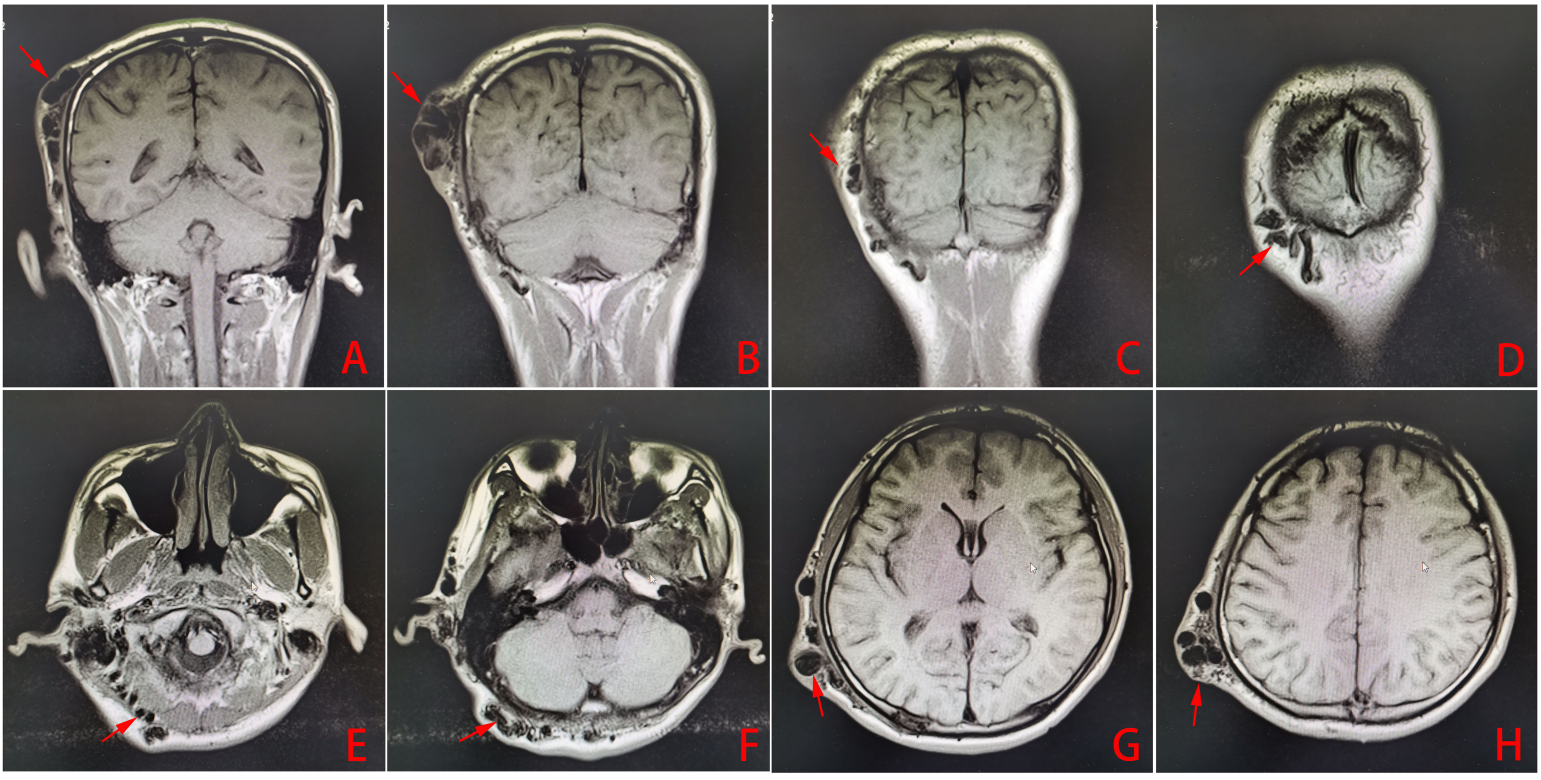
(A–H) Head MRI examination results (the arrows show the abnormal vascular mass of the scalp arteriovenous fistula).
